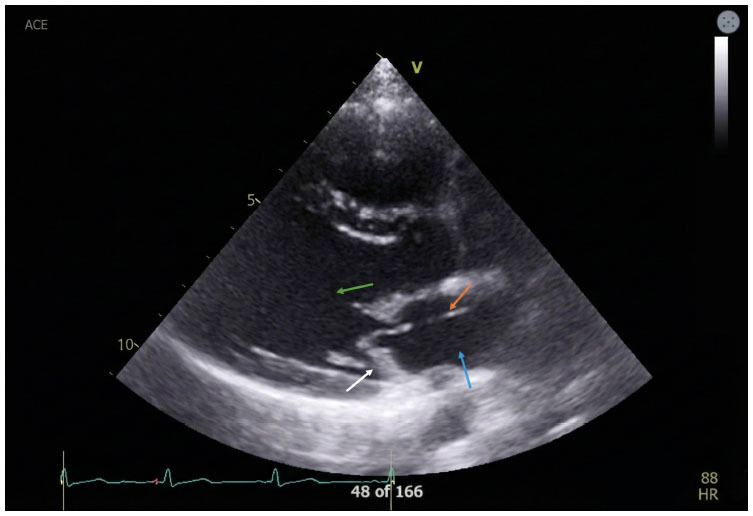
A transesophageal 2D echo with long-axis parasternal view showing the false tendon (orange arrow) traversing the mitral valve (white arrow) from the left atrium (blue arrow) to the left ventricle (green arrow).
